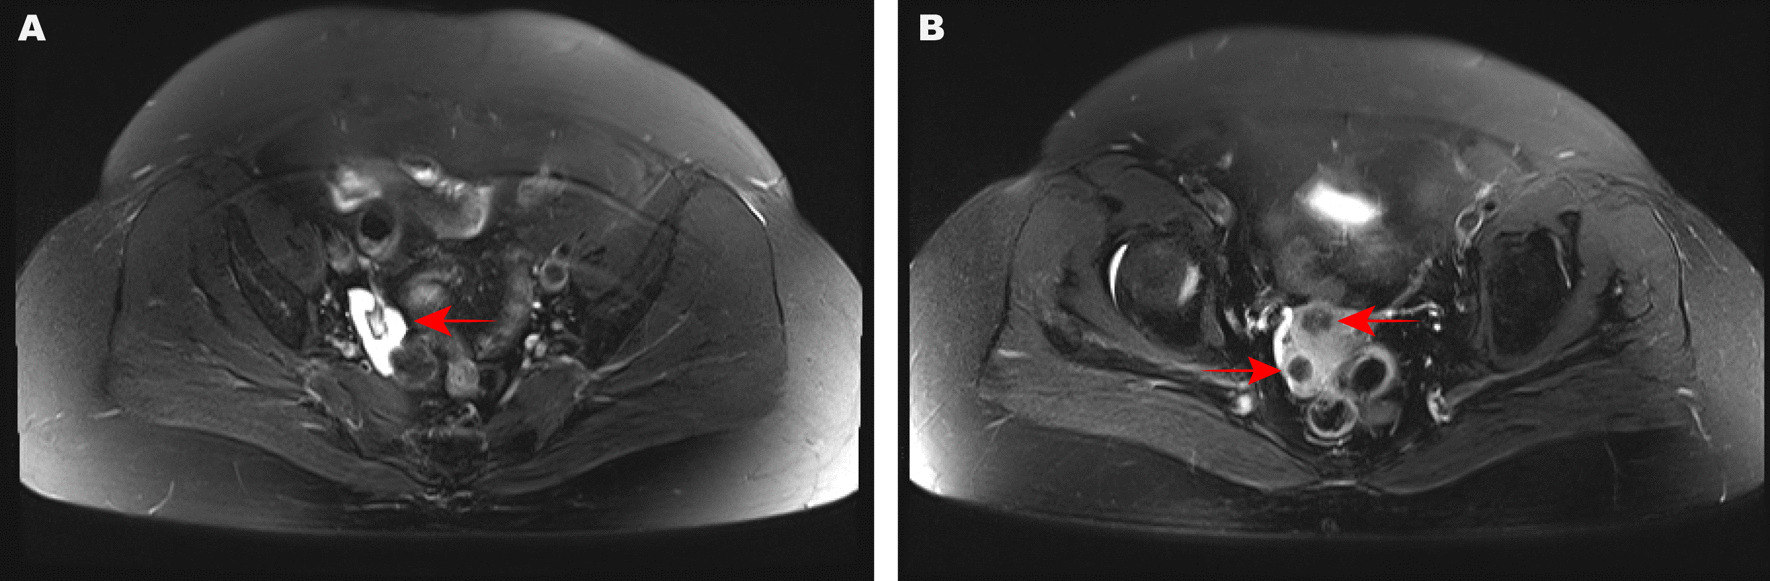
MRI performance. A A mass in the right adnexal area. B Multiple nodules in the uterus
